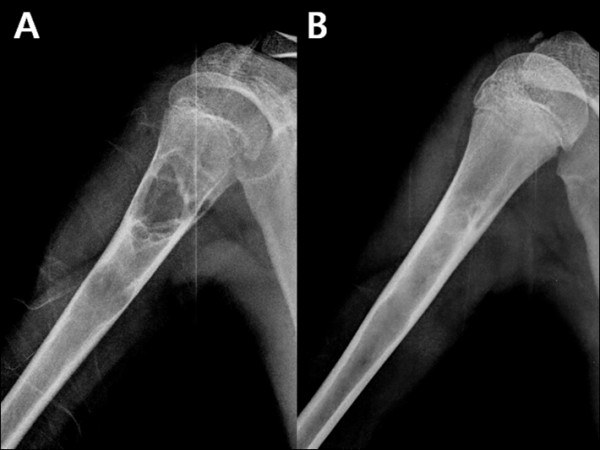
Radiographical cyst healing after injection of DBM. Plain radiographs A) of the right humerus before surgery in a 13-year-old boy with cystic lesion located at the proximal meta-diaphysis B) at postoperative 24 months.
